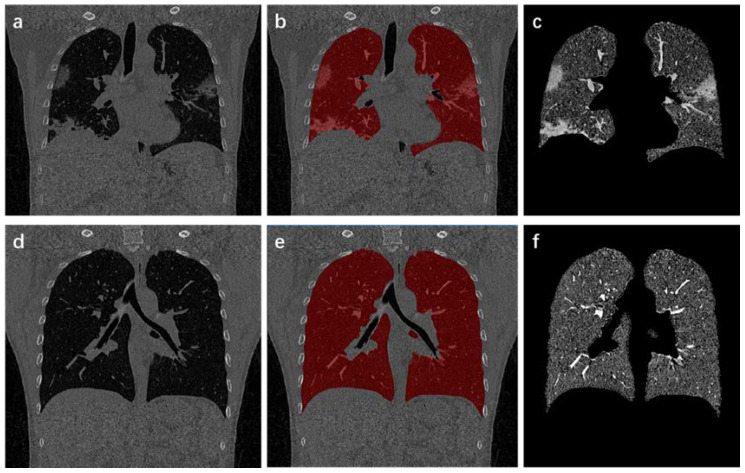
Process and results of lung region extraction. (a,d) are the original CT images; (b,e) show results of lung mask covering the original CT images; (c,f) indicate the extracted lung area.
